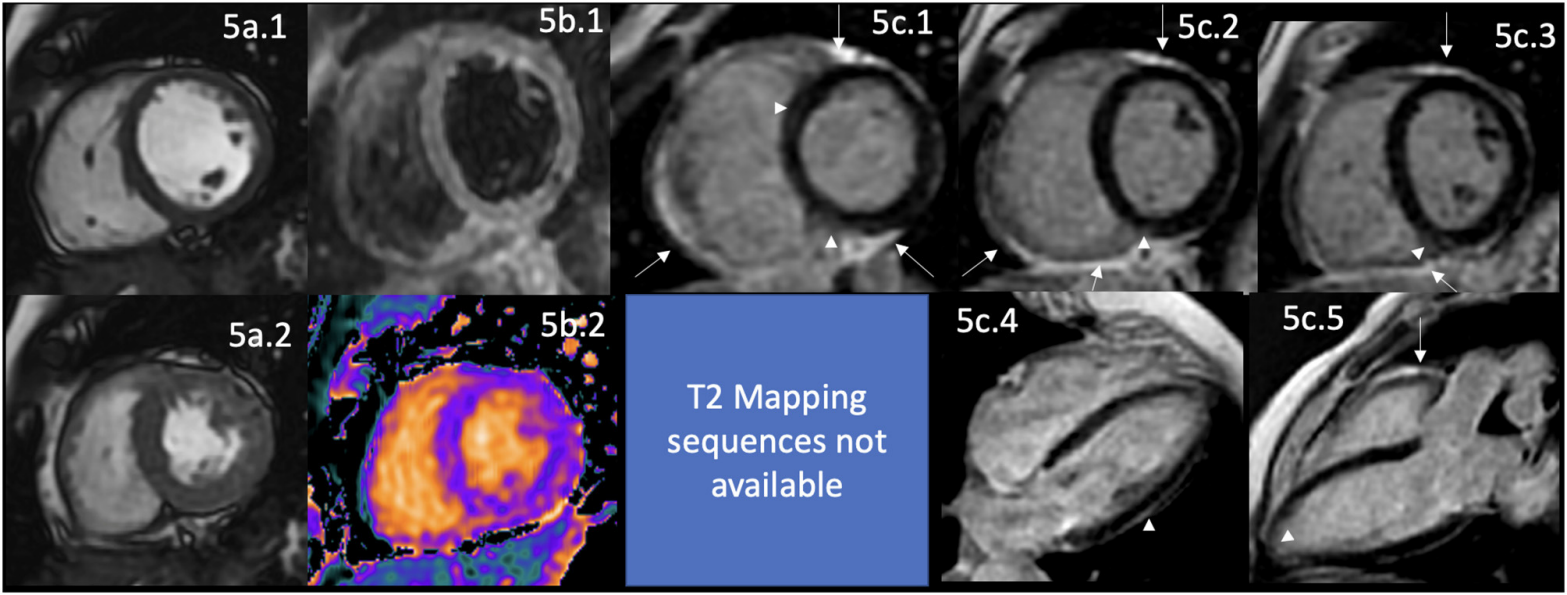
CMR findings in SSc. A panel figure demonstrates varying findings by a comprehensive CMR study at 1.5 Tesla scanner of SS. (a) SSFP sequence still cine images in short-axis view at the mid-ventricular level in end-diastole (a.1) and end-systole (a.2) shows normal global and regional functions of both ventricles, LVEF 57% and RVEF 56%. (b.1) T2-W STIR sequence in short-axis view at the mid-ventricular level of normal myocardium and myocardial/skeletal muscle ratio. (b.2) T1 mapping with a native T1 of 998 ms (mildly increase) and a calculated ECV of 32% (mildly increase). (c.1–5) LGE PSIR sequence. (c.1–3) Short-axis views at basal (c.1), mid (c.2), and apical (c.3) levels, and long-axis views in 4-chambers (c.4) and 3-chambers (c.5). The arrowheads in these images show areas of focal fibrosis as mid-wall LGE of the anteroseptal segment in (c.1) and the subepicardium of the inferior segment in (c.1–3) in the basal and mid anterolateral segments in (c.4) and subendocardial in the LV apex in (c.5). In addition, the same set of images shows LGE of the pericardium without effusion (arrow), suggestive of pericardial inflammation. CMR, cardiovascular magnetic resonance; SS, systemic sclerosis; SSFP, steady-state free precession; LVEF%, left ventricular ejection fraction; RVEF%, right ventricular ejection fraction; T2-W STIR, T2-weighted short-tau inversion recovery; ECV, extracellular volume; LGE, late gadolinium enhancement; PSIR, phase-sensitive inversion recovery.
